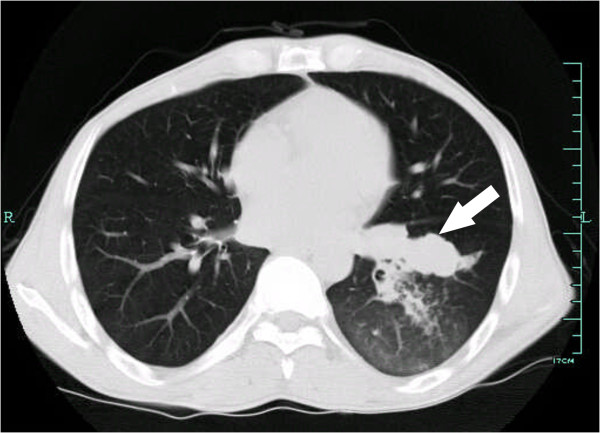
Thoracic computed tomography 2 years after living donor liver transplantation. The white arrow shows a nodular in the hilum of the left lung with the patchy shadow indicating pulmonary infection.
